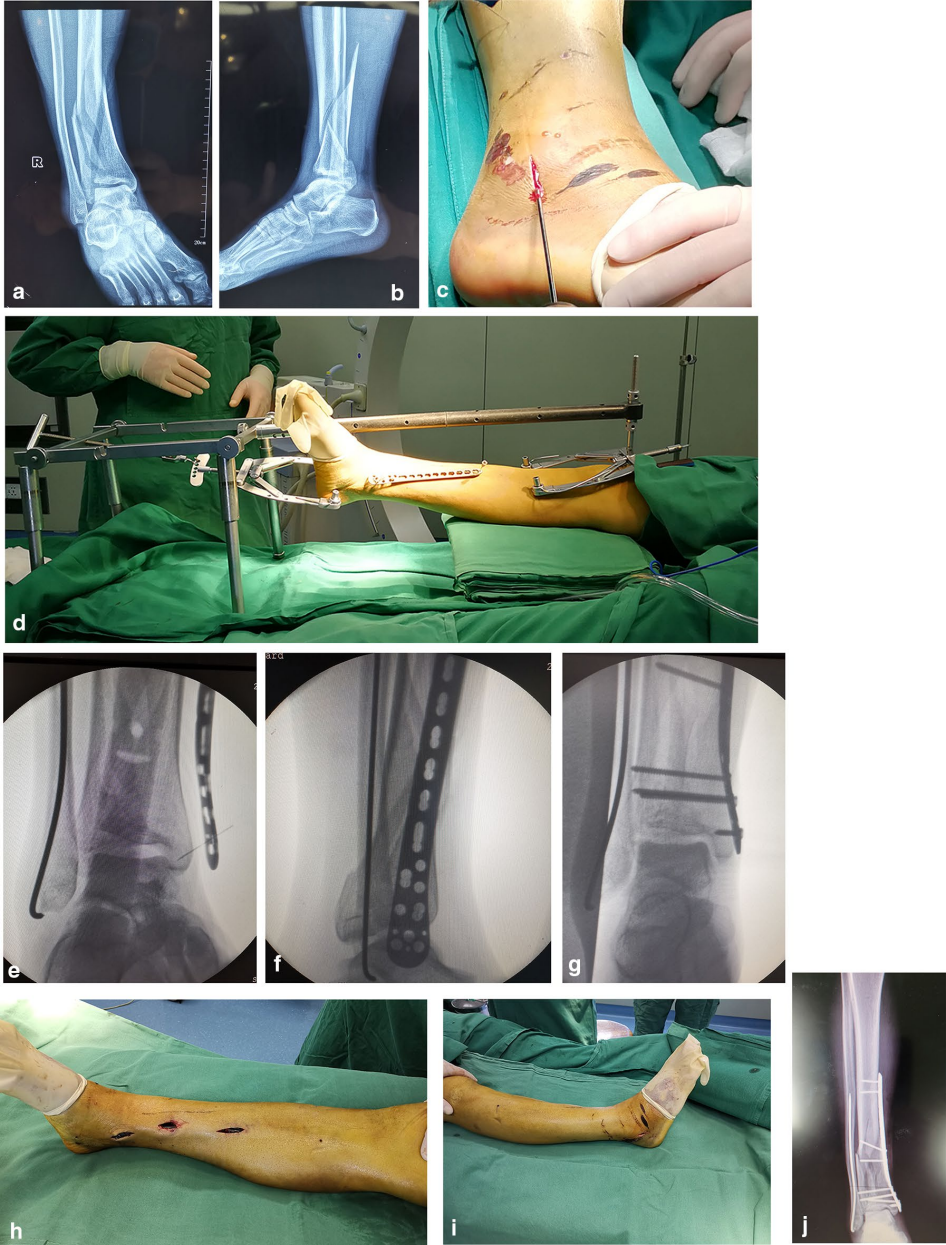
Fixation with MIPO technique. (a) and (b) The anteroposterior and lateral X-rays of a pilon fractures. (c) The fibular was fixation firstly by a 2.5 mm diameter Kirschner wire. (d) The syringe needles were used to locate the position of the plate to achieve minimally invasive incision. (e), (f) The plate was adjusted to the best position under fluoroscopy. (g) Intraoperative fluoroscopy showed that excellent fracture reduction was obtained. The surgical incision was shown in (h) and (i). (j) The plate was fixation with MIPO technique.
